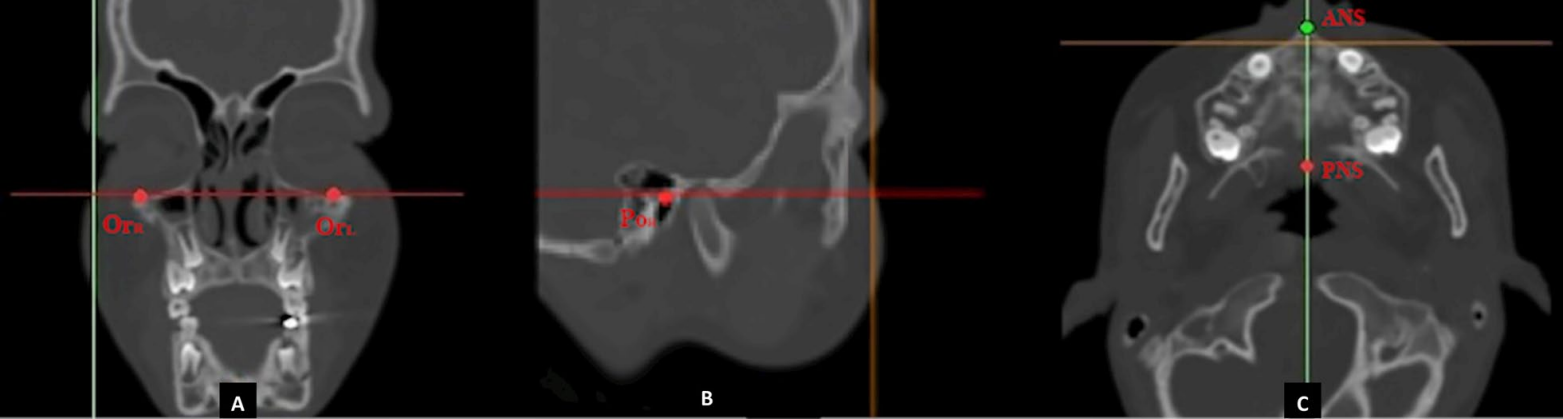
CT image slices of the orientation landmarks: Coronal CT image showing the right orbital Or_R_ and left orbital Or_L_ (a). Sagittal view showing right Porion Po_R_ (b). Axial view showing anterior nasal spine ANS and posterior nasal spin PNS (c)
